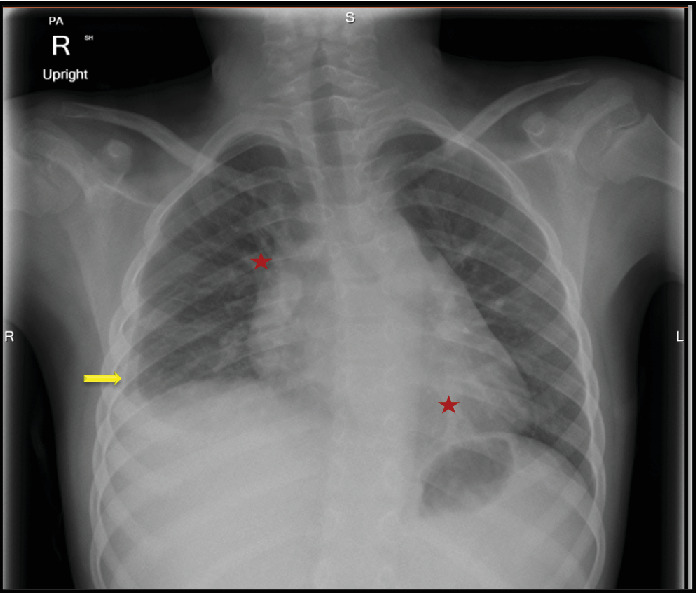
The PA view of the chest X-ray revealed decreased volume in the right lung, accompanied by mild perihilar pulmonary congestion and reticular opacification (red stars). The left lung appears adequately expanded, exhibiting subsegmental streaky atelectasis in the left retrocardiac area (red stars). Mild right basal and lamellar pleural effusions obliterating the right costophrenic angle (yellow arrow) were observed.
